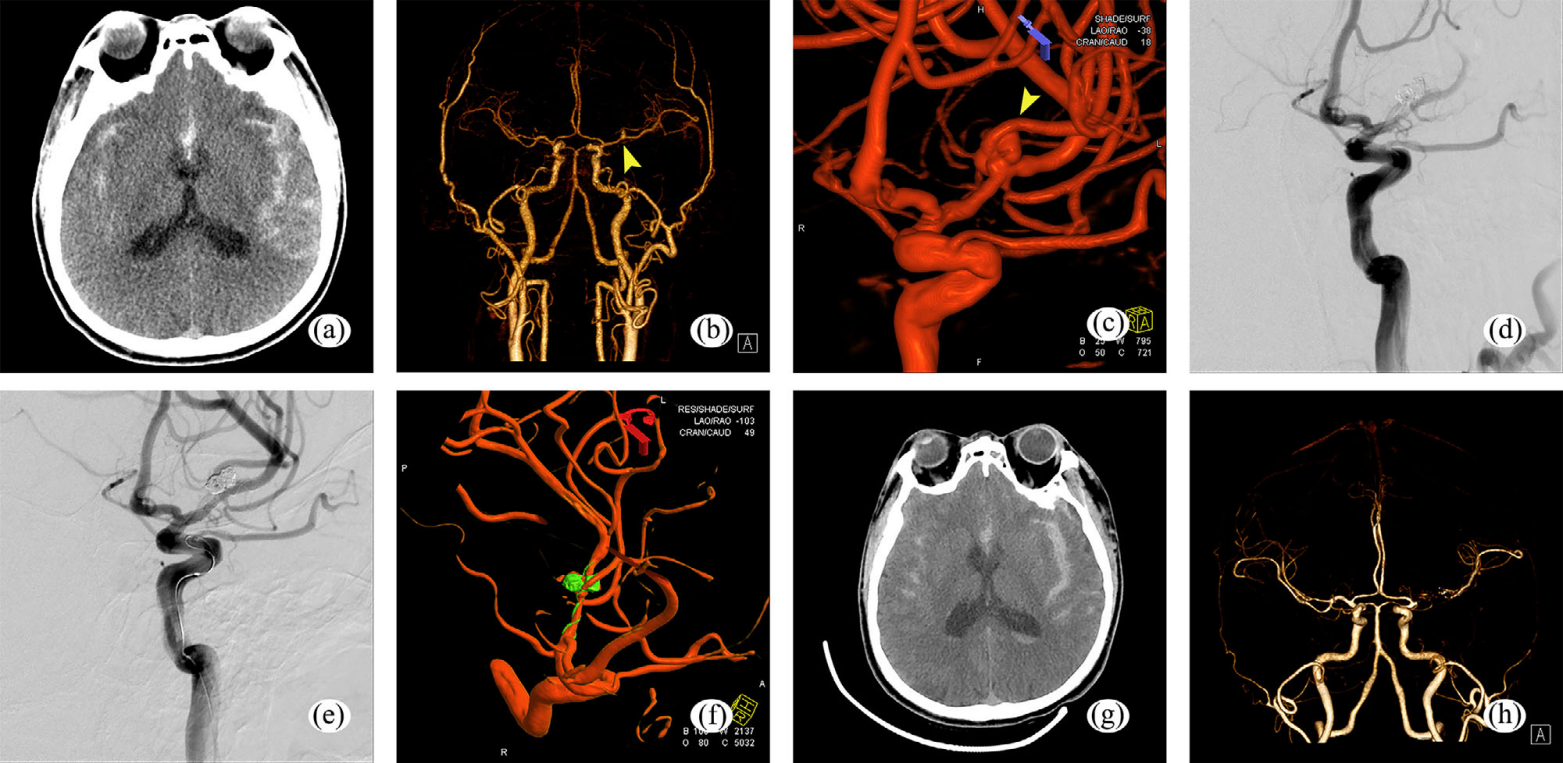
Subarachnoid hemorrhage due to a ruptured aneurysm on segment M1 of the left MCA. (a,b) Brain CTA scan indicating a subarachnoid hemorrhage resulting from a ruptured aneurysm on segment M1 of the left MCA. (c) Cerebral angiography performed to clarify the aneurysm in the M1 segment of the left MCA. (d) Acute thrombosis of the M2 branch of the left MCA was considered when it could not be visualized during the stent‐assisted embolization. (e) Visualization of MCA branches after repeated tirofiban injections and intravenous pumping. (f) MCA branches are clearly visualized on cerebral angiography with 3D imaging. (g,h) Brain CTA performed on the sixth day after surgery showed a distinct bifurcation of the left MCA and clear branching vessels distal to the bifurcation.MCA, middle cerebral artery.
